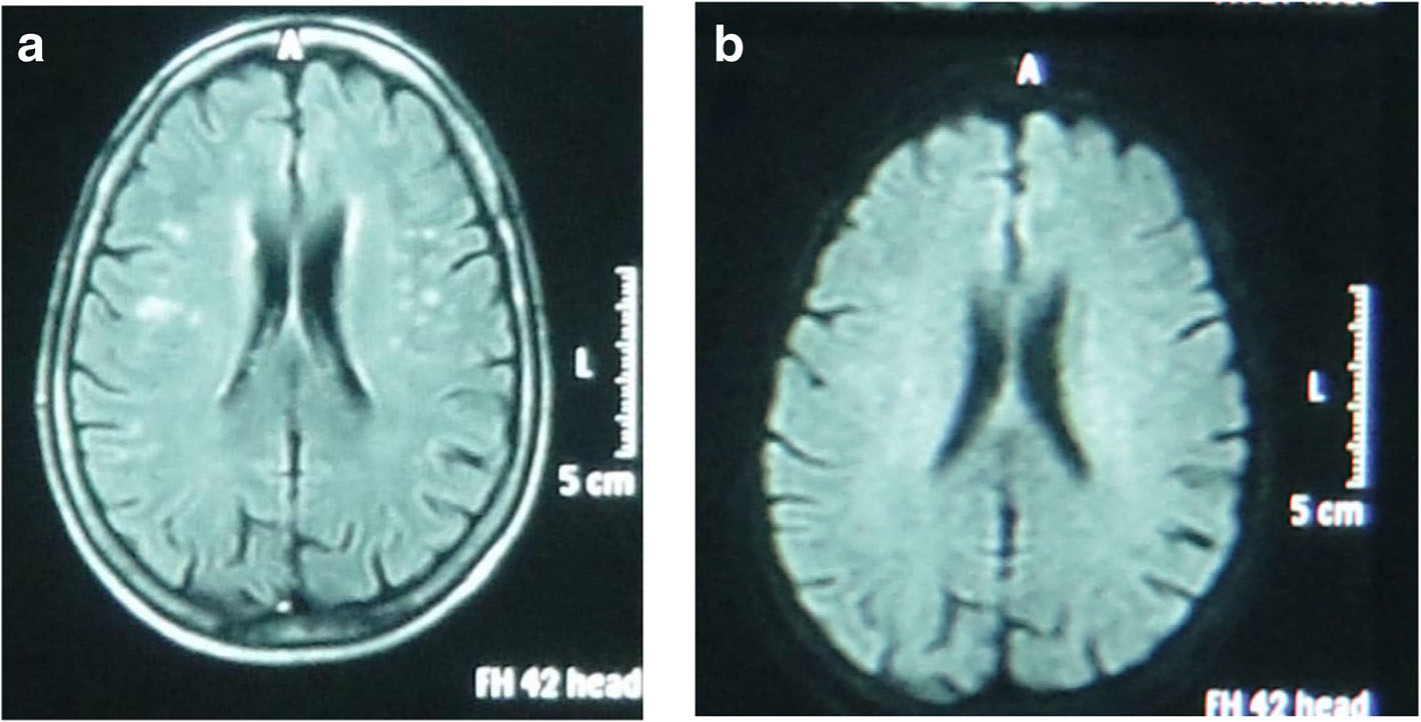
Brain MRI for a female patient aged 42 years who suffered from SSc, disease duration: 5 years. a FLAIR-MRI shows multiple rather well-defined hyperintense. signal foci seen in the deep periventricular white matter of both parietal regions with no comparable signal abnormality on DWI (b) suggesting late subacute to chronic ischemic lesions
